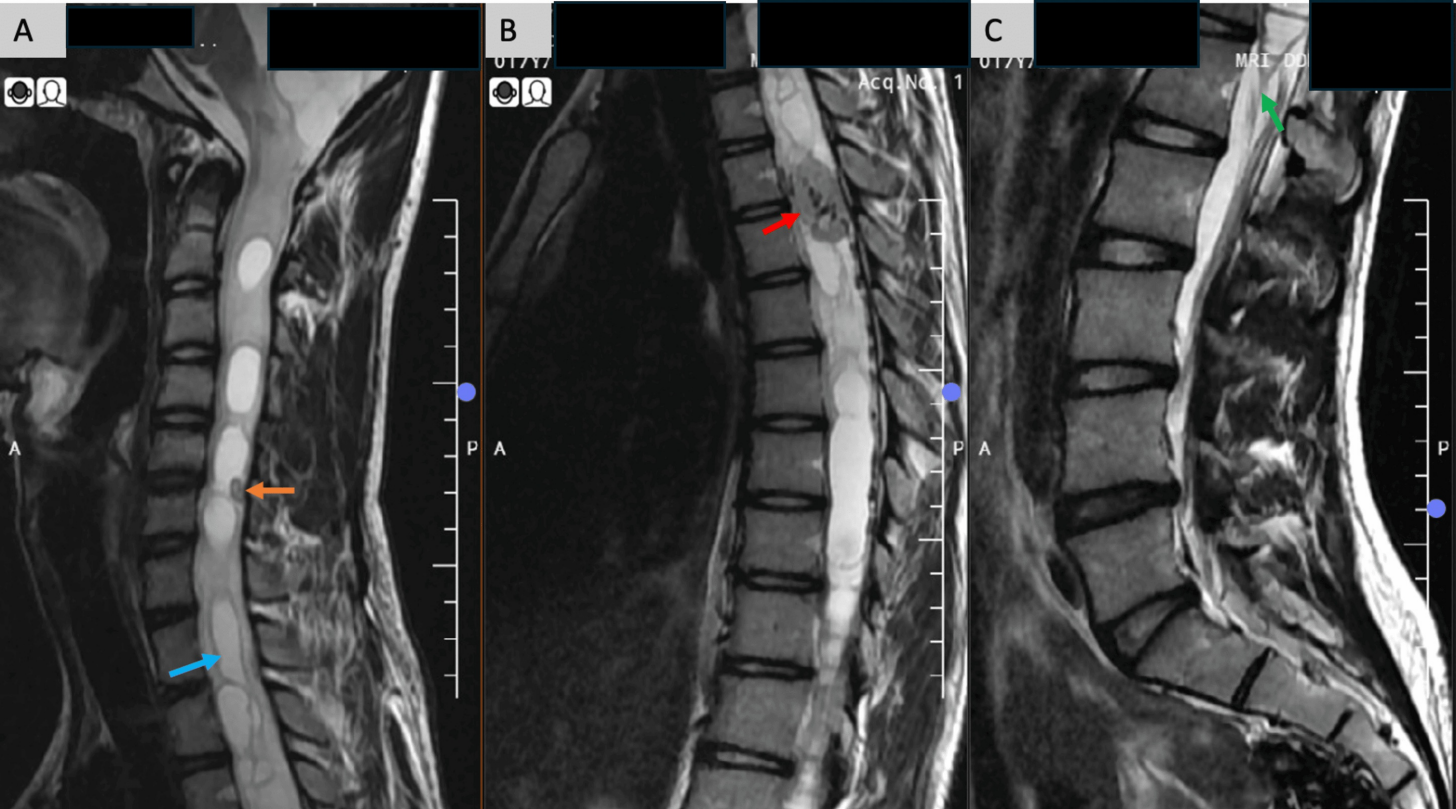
MRI sagittal T2-weighted image (A) Extensive syrinx formation (blue arrow) was seen extending superiorly up to the cervico-medullary junction, and a tiny T2-hypointense focus at C5-C6 level (orange arrow); (B) T2-hypointense intramedullary lesion with internal flow voids noted at the D4-D5 level (red arrow); (C) Inferior extension of the syrinx noted extending up to the level of conus (green arrow).
